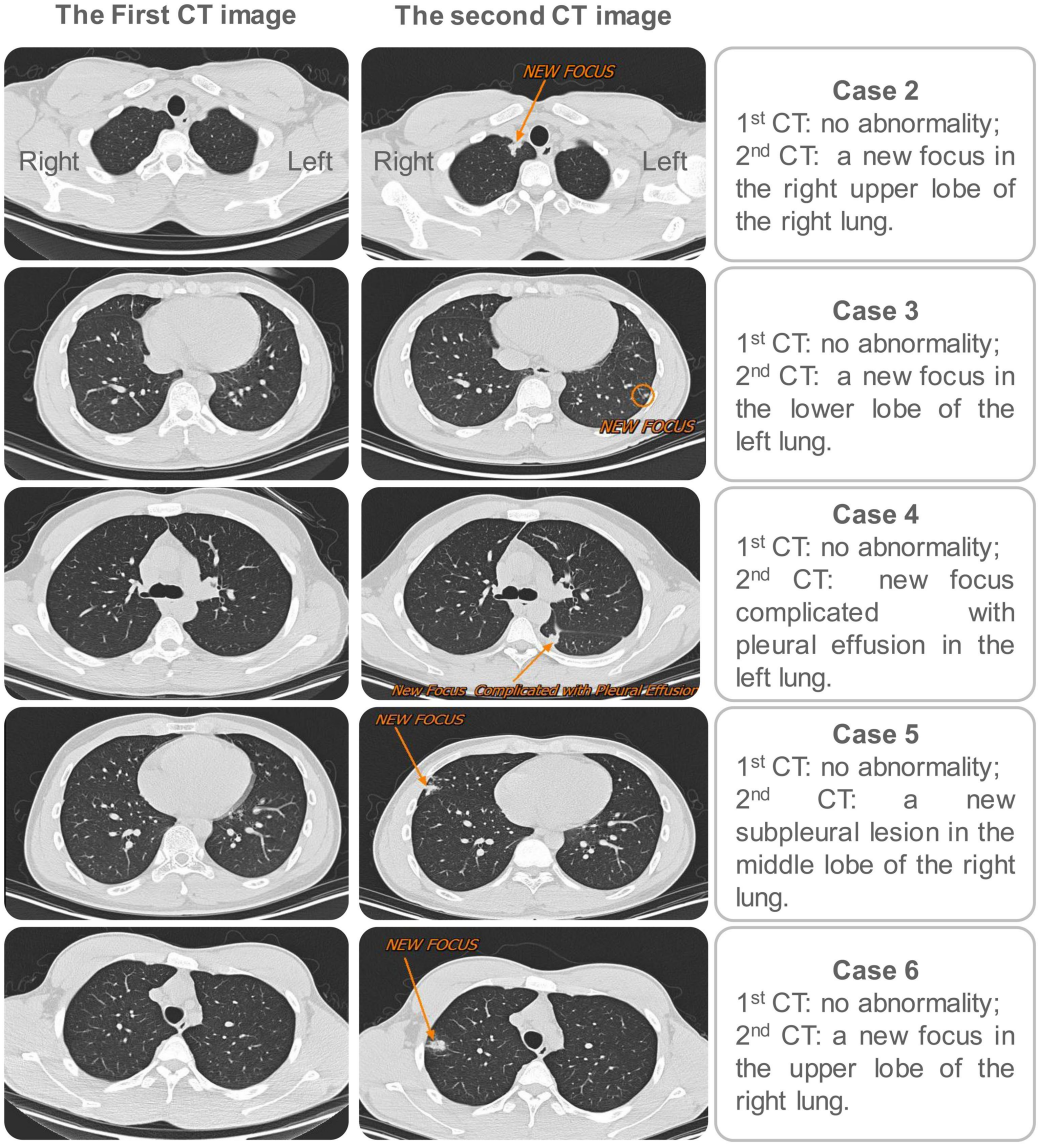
Comparison of the first and second screenings of chest CT for the five secondary cases. In the first screening, no abnormalities were found in the chest CT for the 5 cases. Three months later, the second screening of chest CT was performed, and new lesions were observed in the lungs of all five cases. Left: the first CT image for each case; Right: the second CT image for each case.
